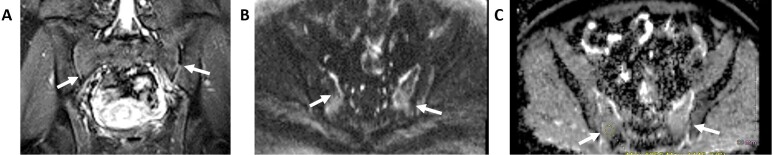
Seronegative psoriasis related sacroiliitis (arrows). Coronal STIR (A) shows trace fluid in SI joints without erosive changes. Axial DWI (B) and ADC (C) images show significant increased signal on both DWI and ADC along subchondral surfaces of both SI joints consistent with active sacroiliitis. ADC, apparent diffusion coefficient; DWI, diffusion-weighted imaging; SI, signal intensity; STIR, short tau inversion recovery.
